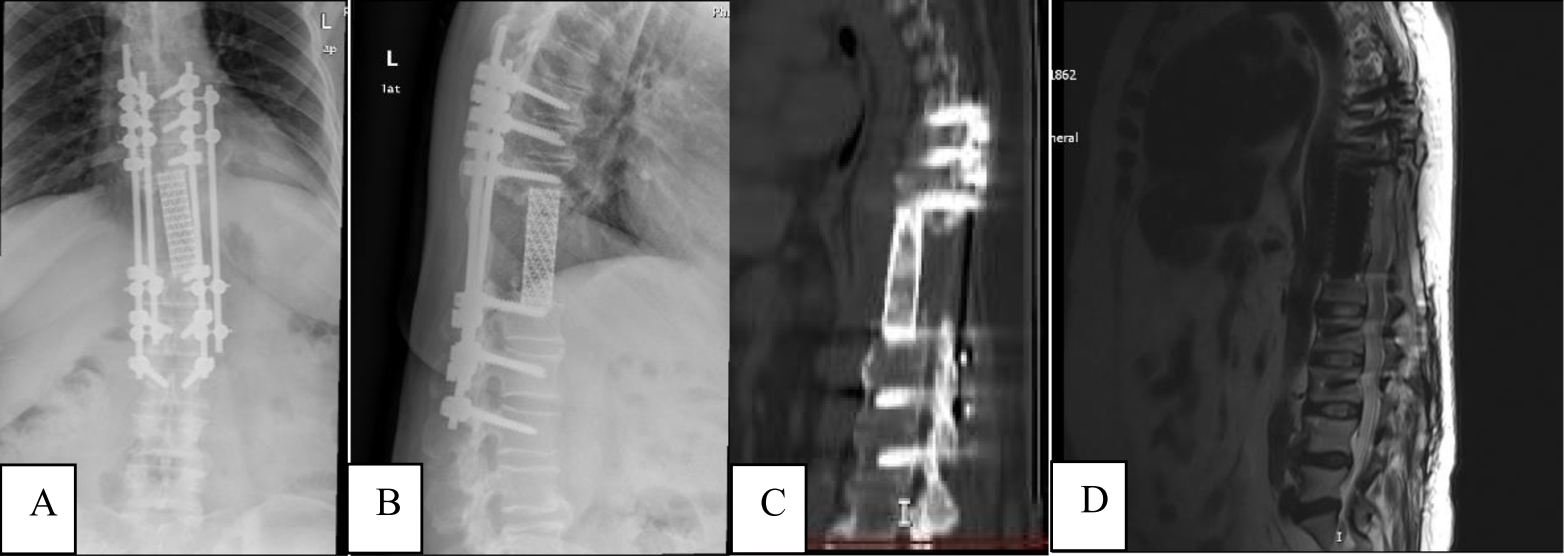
The images of radiograph, CT and MRI for thoracolumbar spine at 32 months after TES. (a) Anteroposterior and (b) lateral radiographs; (c) three-dimensional CT scan; and (d) MRI of the thoracolumbar spine showing a stable construct, biological fusion, and the absence of local recurrence
